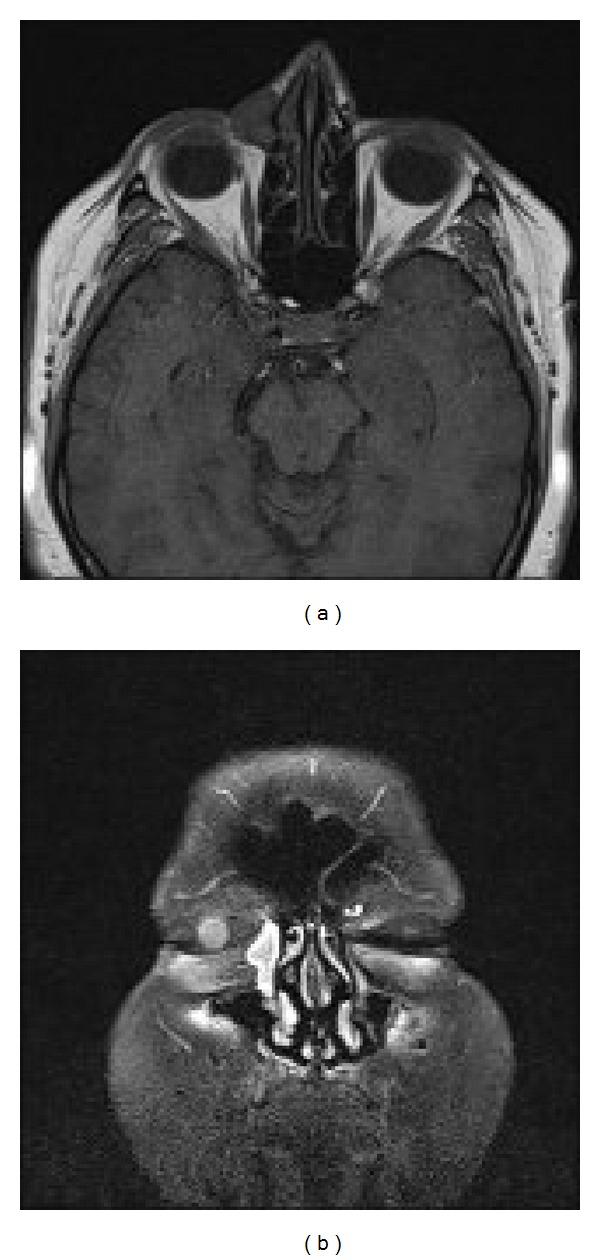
In MR imaging with axial and coronal contrast, it is observed that the right lacrimal sac is quite a lot bigger compared to the left eye and differentiates from the adjacent tissues by way of contrast involvement.
